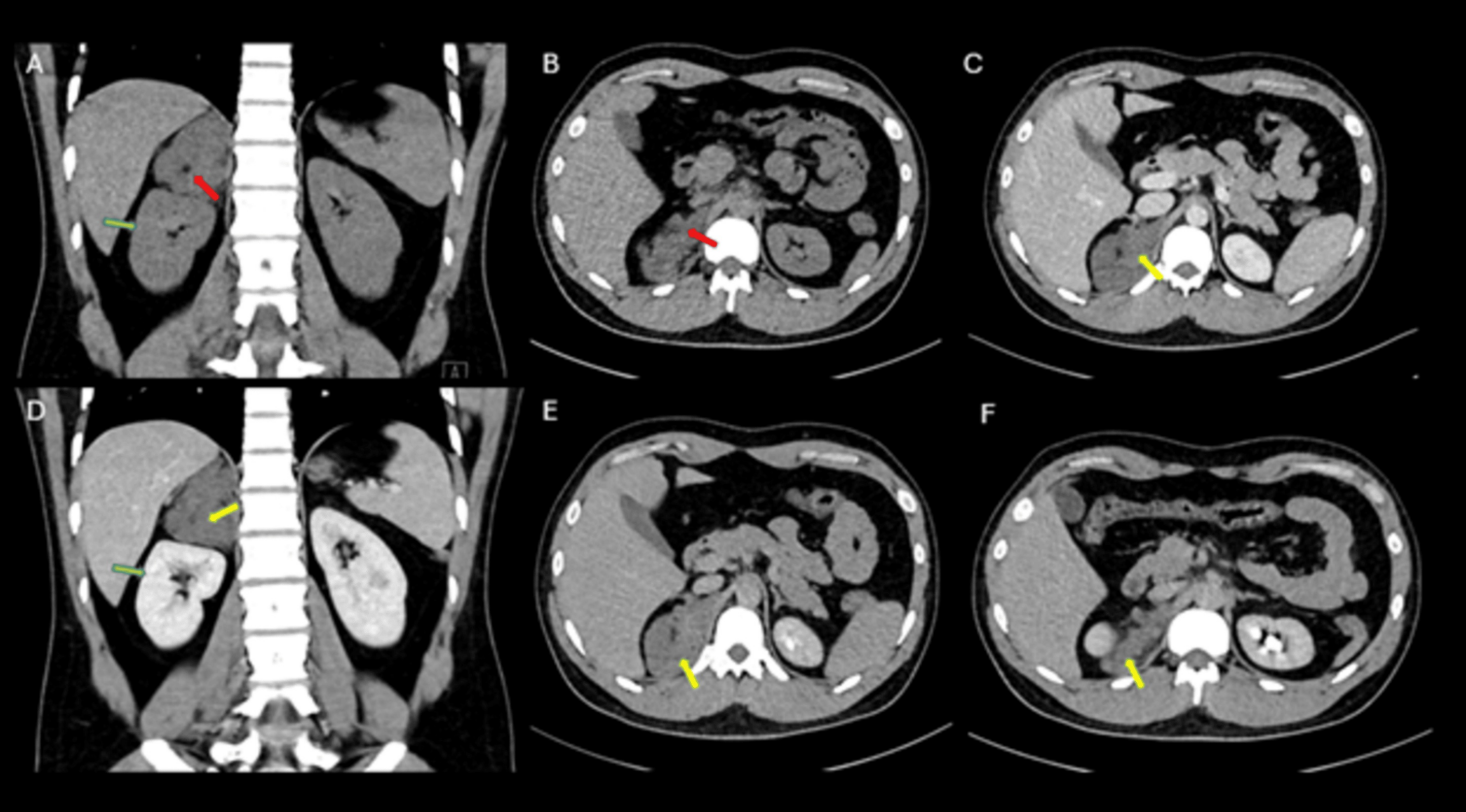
CT Abdomen and pelvis with IV contrast plain. A) coronal; B) axial, venous phase; C) axial; D) coronal, delayed phase; E) and F) showing right adrenal soft tissue mass with lobulated contour mostly arising from the medial limb (red arrows). There are intra-lesional macroscopic fat components with no calcification seen. On post-contrast images, there is progressive contrast enhancement (yellow arrows). The right kidney is displaced inferiorly by the adrenal mass with no invasion (green arrows).
